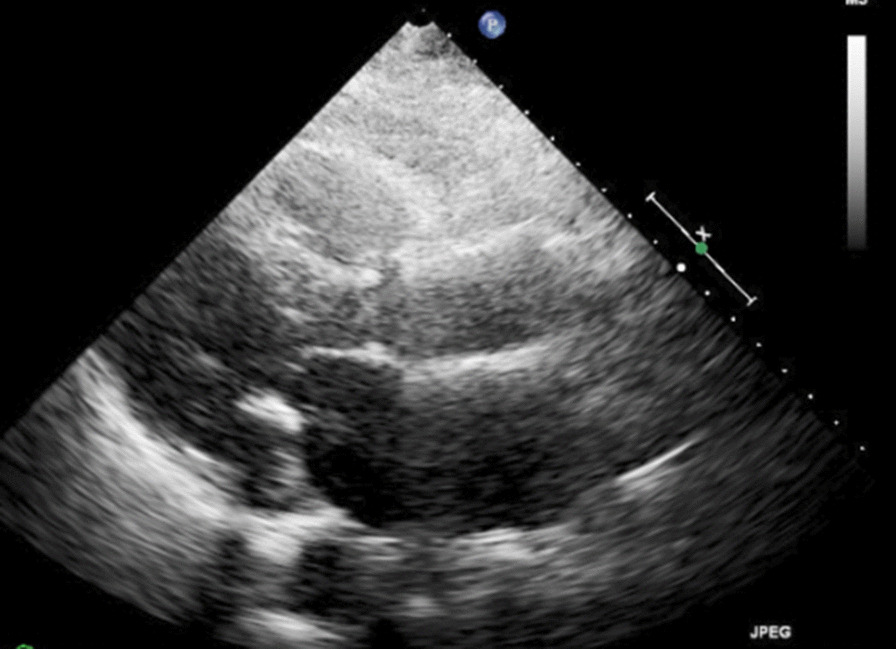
Transthoracic echocardiogram in parasternal long axis view showing calcified appearance of mitral and aortic annuli with nonspecific thickening of the interventricular septum
